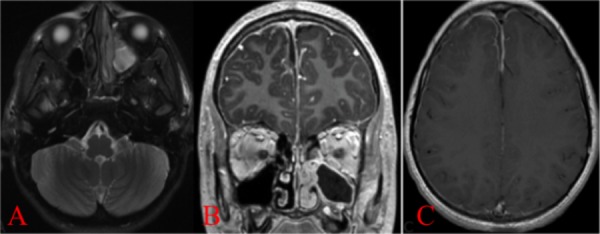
A) Axial T2 Magnetic resonance imaging (MRI) showing involvement of the paranasal sinuses. B) and C) axial and coronal gadolinium-enhanced MRI showing less than 2 mm subdural empyema, an extension from the sinusitis
